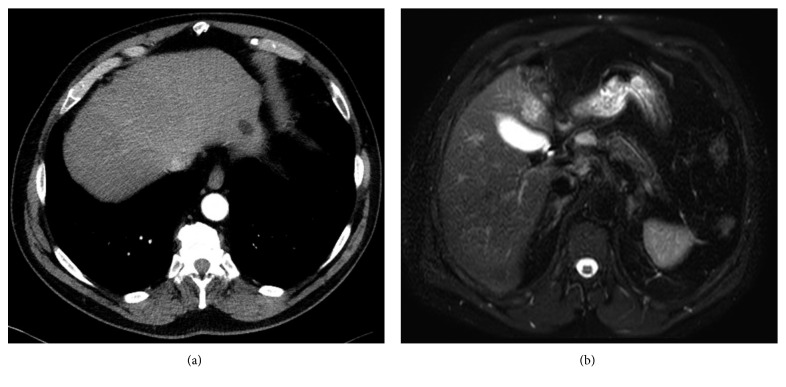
MRI abdomen showing 1.8 cm liver cyst (a) and 1.3 cm ovoid liver lesion (b).
